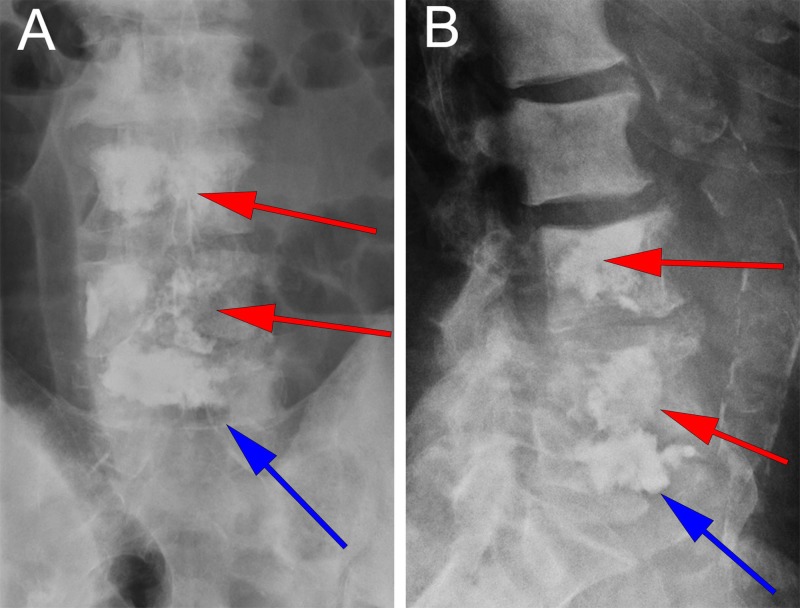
Postoperative lumbar X-Ray Anterior (A) and lateral (B) views demonstrating polymethylmethacrylate (PMMA) cement in the third and fourth lumbar vertebral bodies (L3 and L4, respectively). No extravasation of cement into the spinal canal is noted with normal post-operative changes. Red arrows delineate cement within the L3 and L4 vertebral bodies. Blue arrows delineate extravasated cement from the L4 vertebral body into the L4-L5 disc space.
